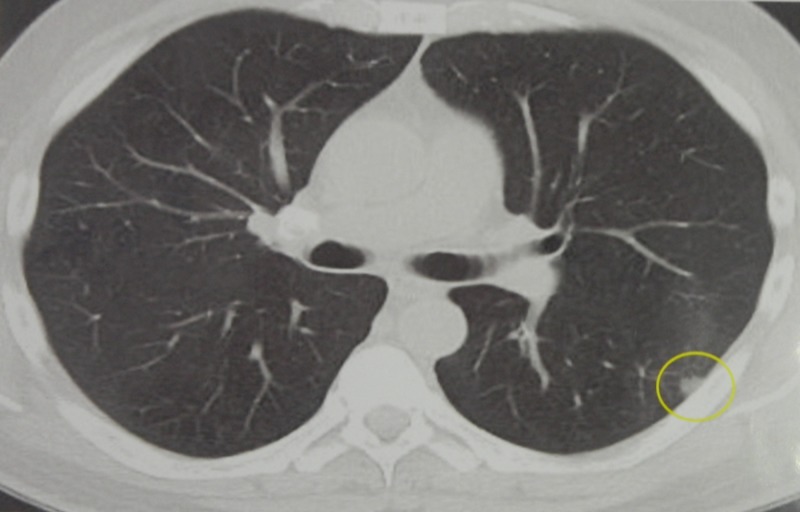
Axial Computed Tomography of a Small Pulmonary Nodule. An axial computed tomography study demonstrates a ground-glass nodular opacity in the left lower lobe (yellow circle).
